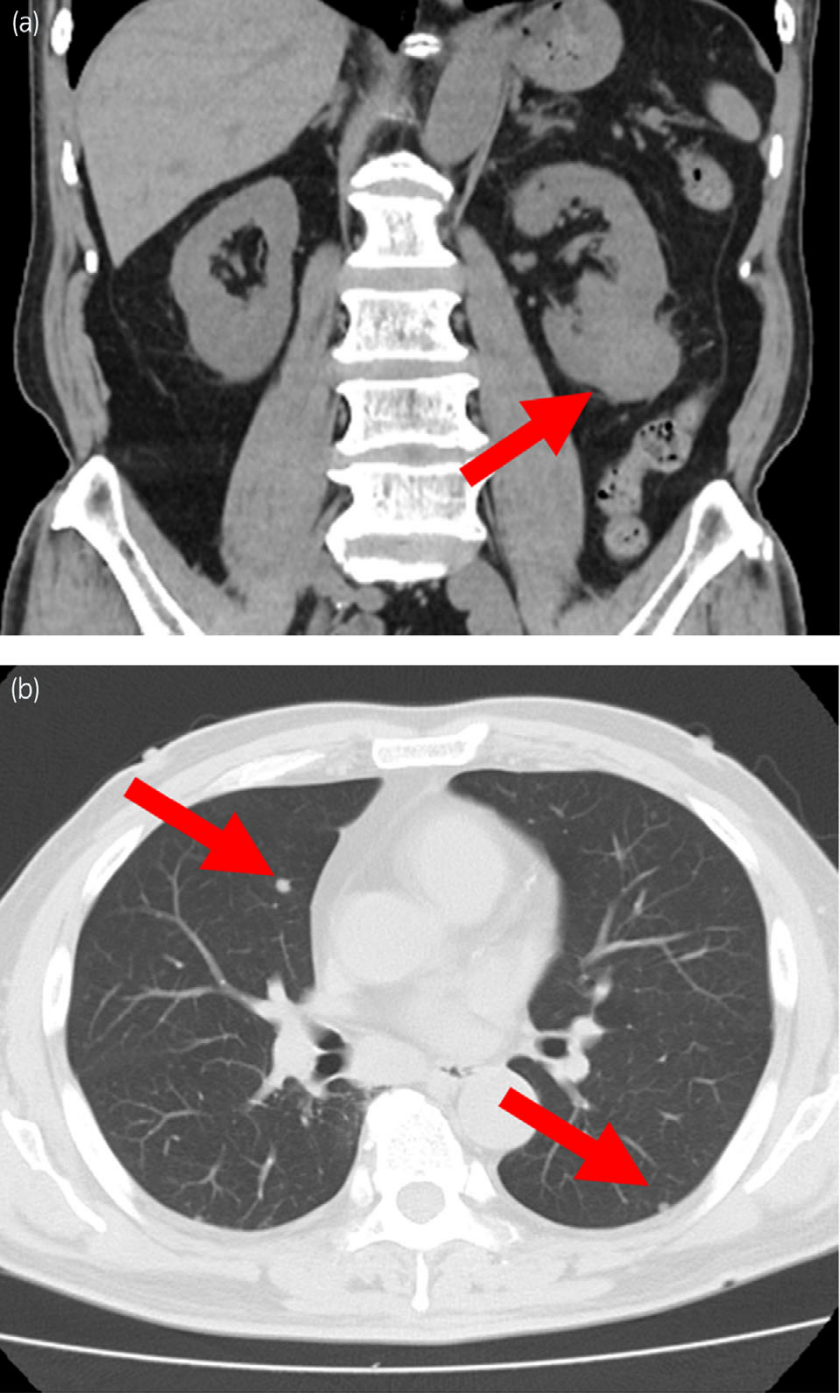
(a) Non‐contrast coronal computed tomography (CT) image showing a tumor measuring 34 mm in diameter in the left upper pole of the kidney. (b) Axial CT image showing multiple lung metastases in both lung fields.
